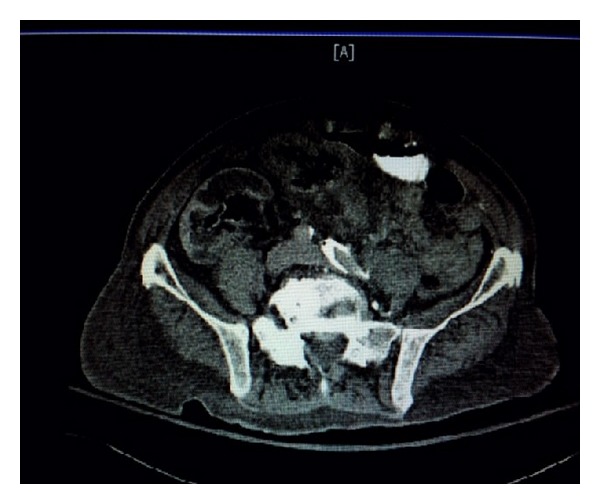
CT scan of the abdomen and pelvis showing an edematous transplanted kidney with air in the intraparenchymal and pelvic regions and the bladder.
